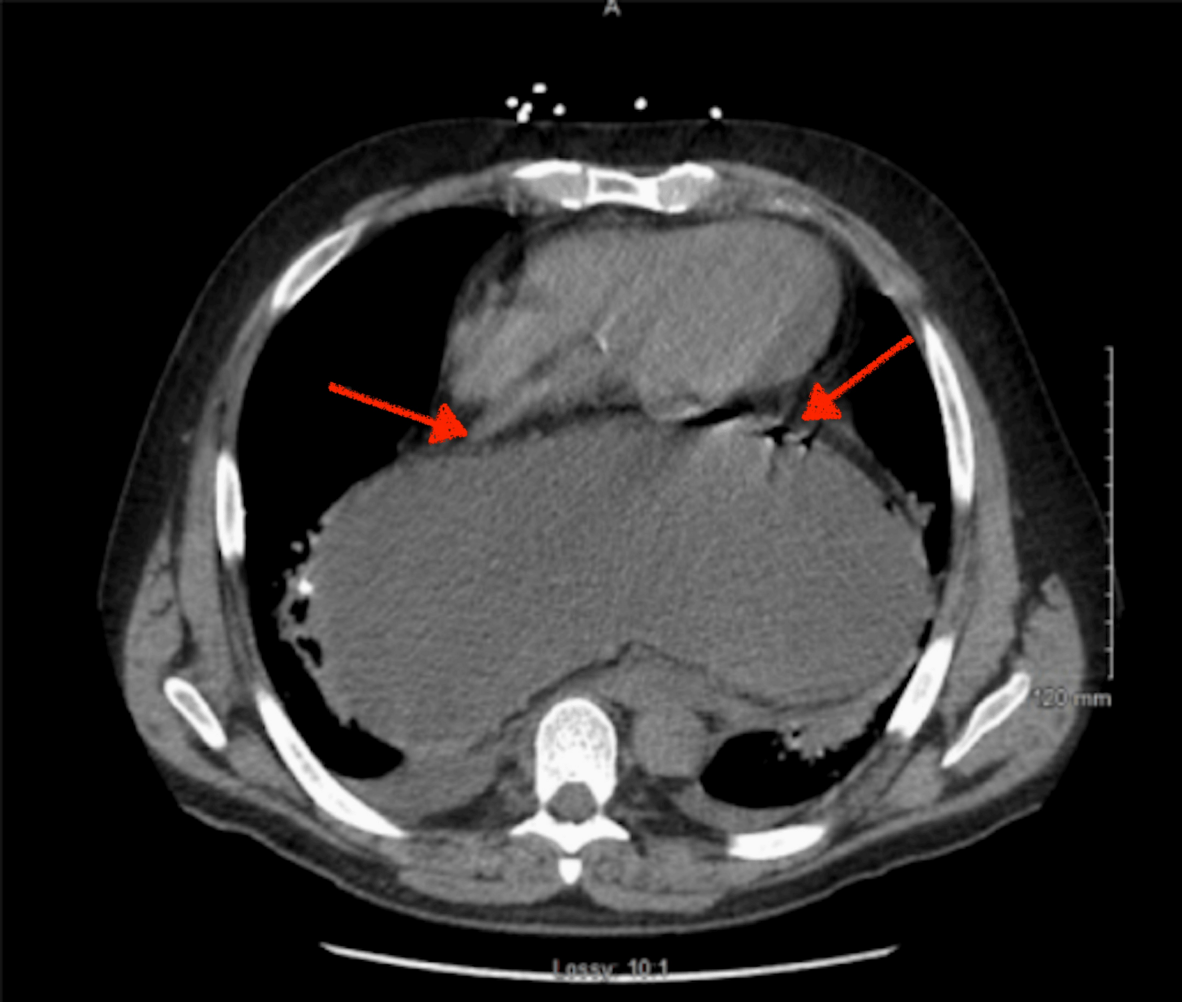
Axial computed tomography demonstrating intrathoracic displacement of the stomach (left arrow) with associated intramural gas within the gastric wall (right arrow). These findings are concerning for gastric ischemia in the setting of mesenteroaxial gastric volvulus and a large hiatal hernia.
